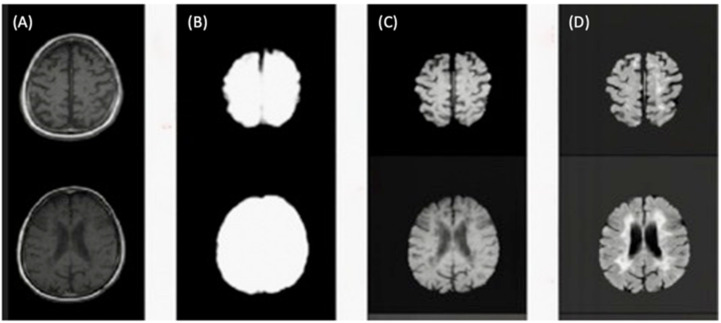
(A) Slices from original T1-w image. (B) Brain mask through skull-stripping using CNN. (C) Brain extraction in T1-w. (D) Brain extraction in FLAIR image.
